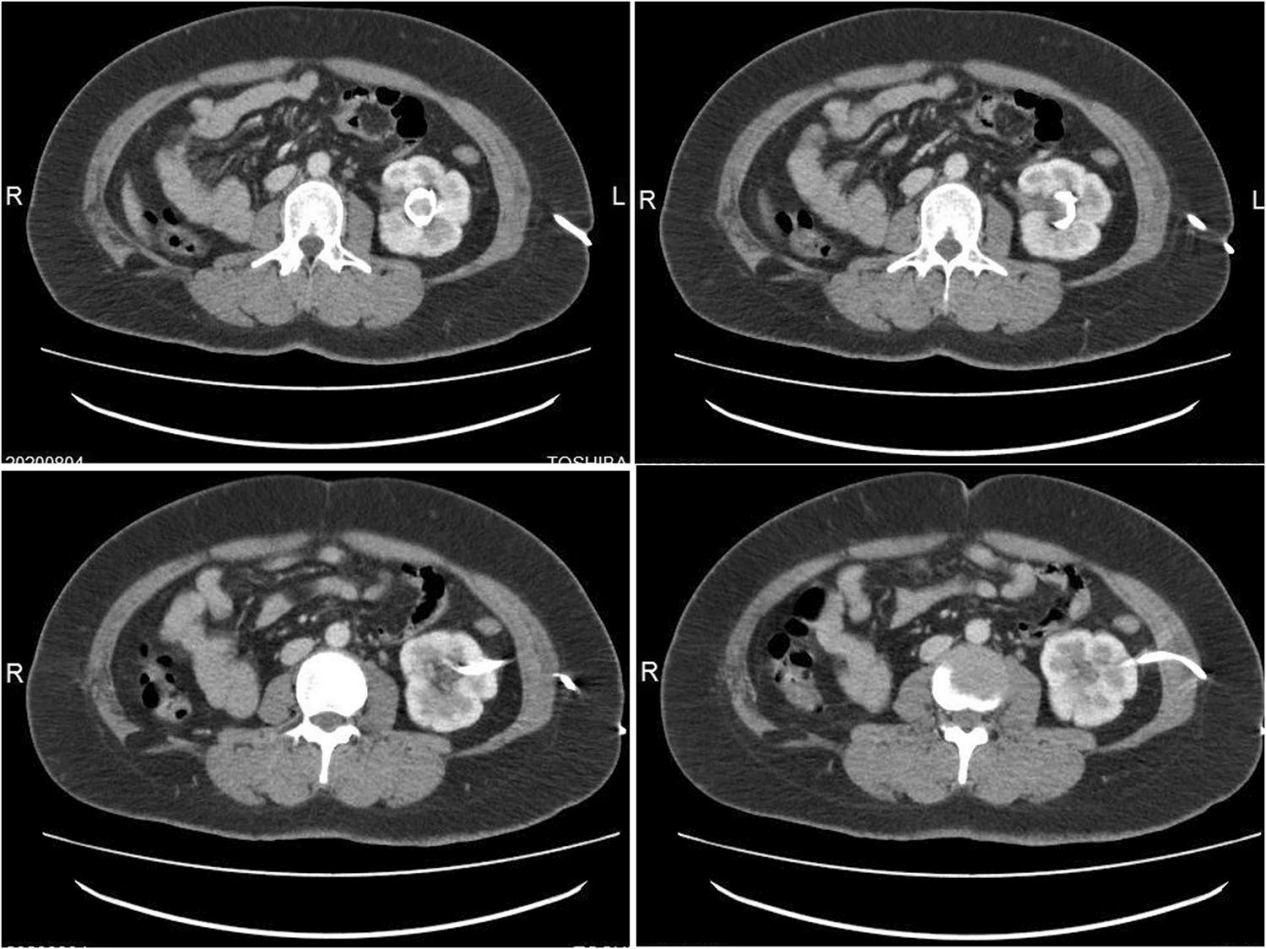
Abdominal CT images showed that the patient’s right kidney was absent, and a drainage tube was located in the left renal pelvis and communicated with the outside of the body
